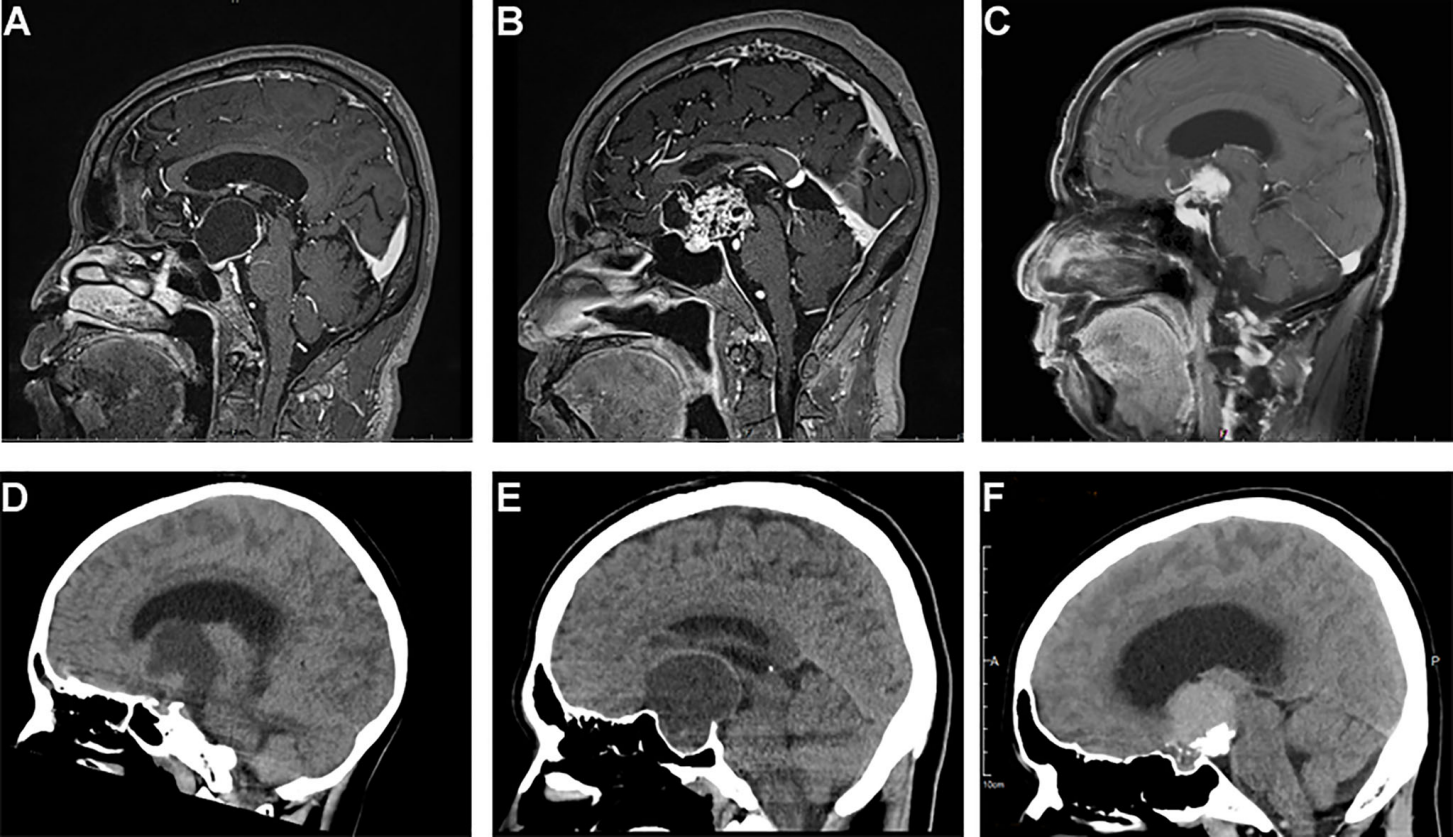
Typical sagittal MRI and CT imaging pictures of craniopharyngiomas. (A–C) MR images, (A) cystic suprasellar–pseudointraventricular PCP, (B) mixed solid cystic infundibulo-tuberal ACP, and (C) solid infundibulo-tuberal PCP. (D–F) CT images, (D) a mixed solid-cystic case of PCP, no calcification; (E) a mixed cystic-solid case of ACP showing peripheral eggshell calcification; and (F) a solid case of ACP, big block popcorn calcification.
